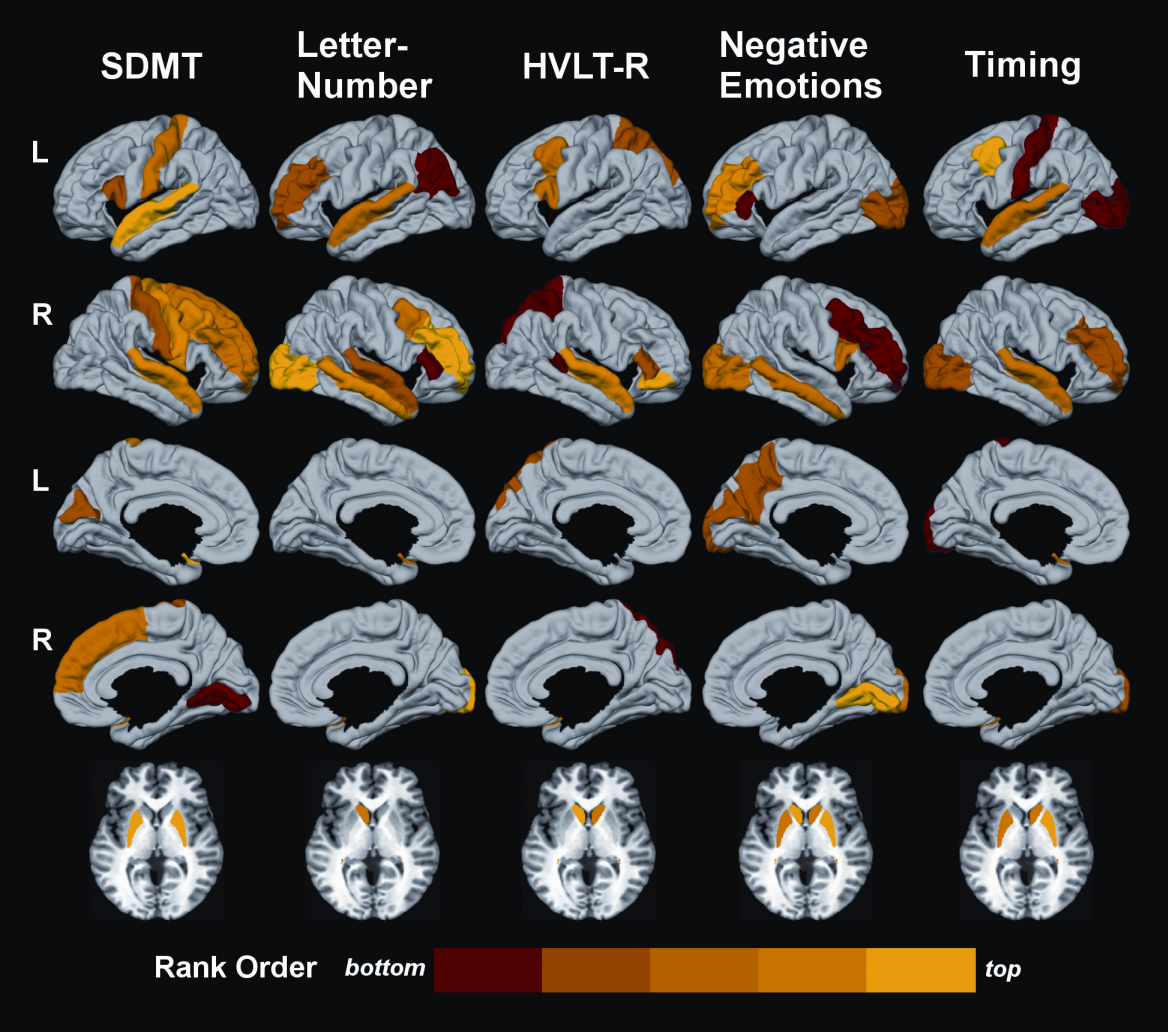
Spatial maps of the top-ranked structural MRI (sMRI) correlates of performance in each cognitive domain. Cortical regions are displayed on the lateral (1st and 2nd rows) and medial (3rd and 4th rows) surfaces of the left (L) and right (R) hemispheres. The basal ganglia are shown at the bottom. The importance of a brain region in correlating with a cognitive measure is color coded on a continuum (red to yellow) according to the rank order of the mean square error (MSE) value for a sMRI variable, where larger MSEs signified greater importance. Yellow signifies a higher rank order of importance than red. Colors on the bar designate variables ranked in the top 20th (yellow) to the bottom 20th (red) percentile of the top-ranked sMRI predictors for each cognitive measure.
